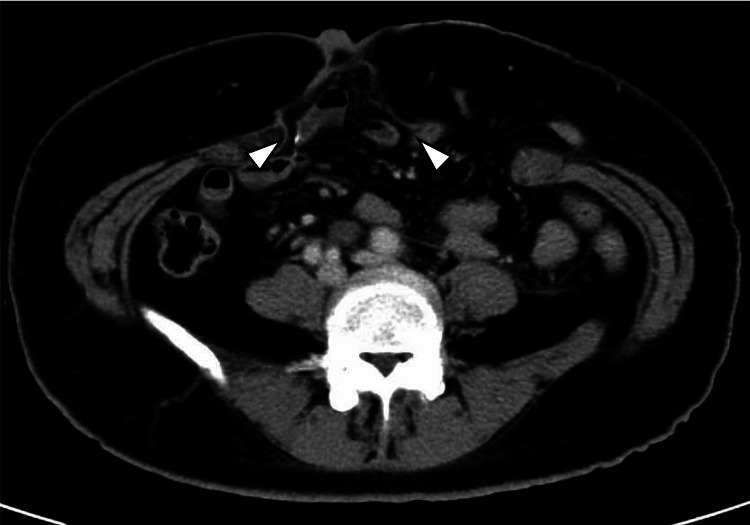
Abdominal midline incisional hernia Contrast-enhanced computed tomography revealed an abdominal midline incisional hernia with an orifice of 15x8.0 cm. Small intestinal prolapses were evident in the hernia (arrowheads).
